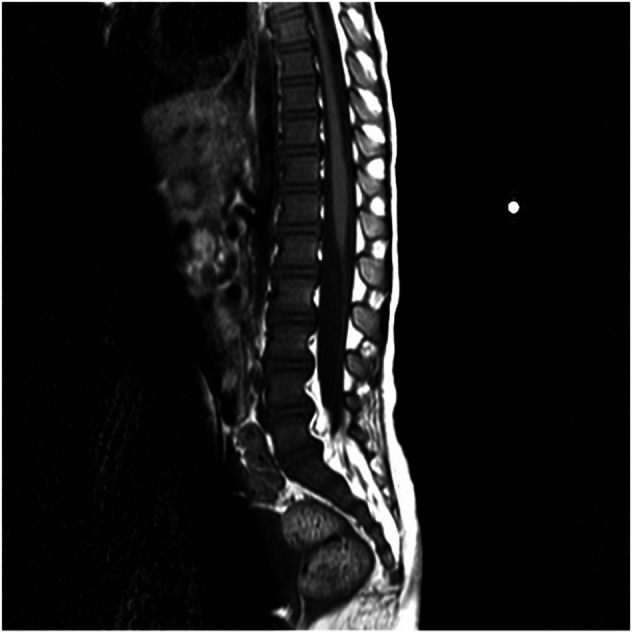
T1-weighted sagittal image of the spinal cord MRI. No abnormal findings were observed.
